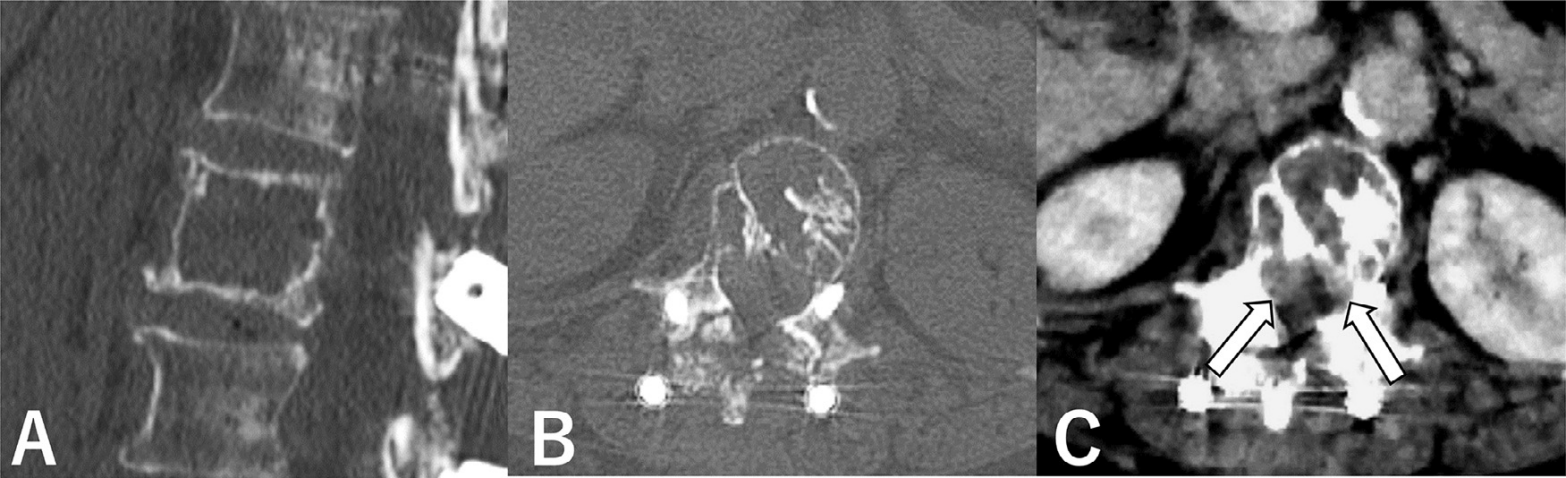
Aggressive vertebral hemangioma involving the L1 vertebral body in a 79-year-old female with lower back pain and symptoms of neurological compression. (A, B) Sagittal and axial CT showing an expansive and osteolytic lesion in the entire vertebral body of L1 with erosion of the posterior vertebral wall. (C) Axial contrast-enhanced CT showing an enhancing lesion involving the entire vertebral body. The lesion extends into the anterior epidural space (arrows), causing compression of the cauda equina. CT, computed tomography.
